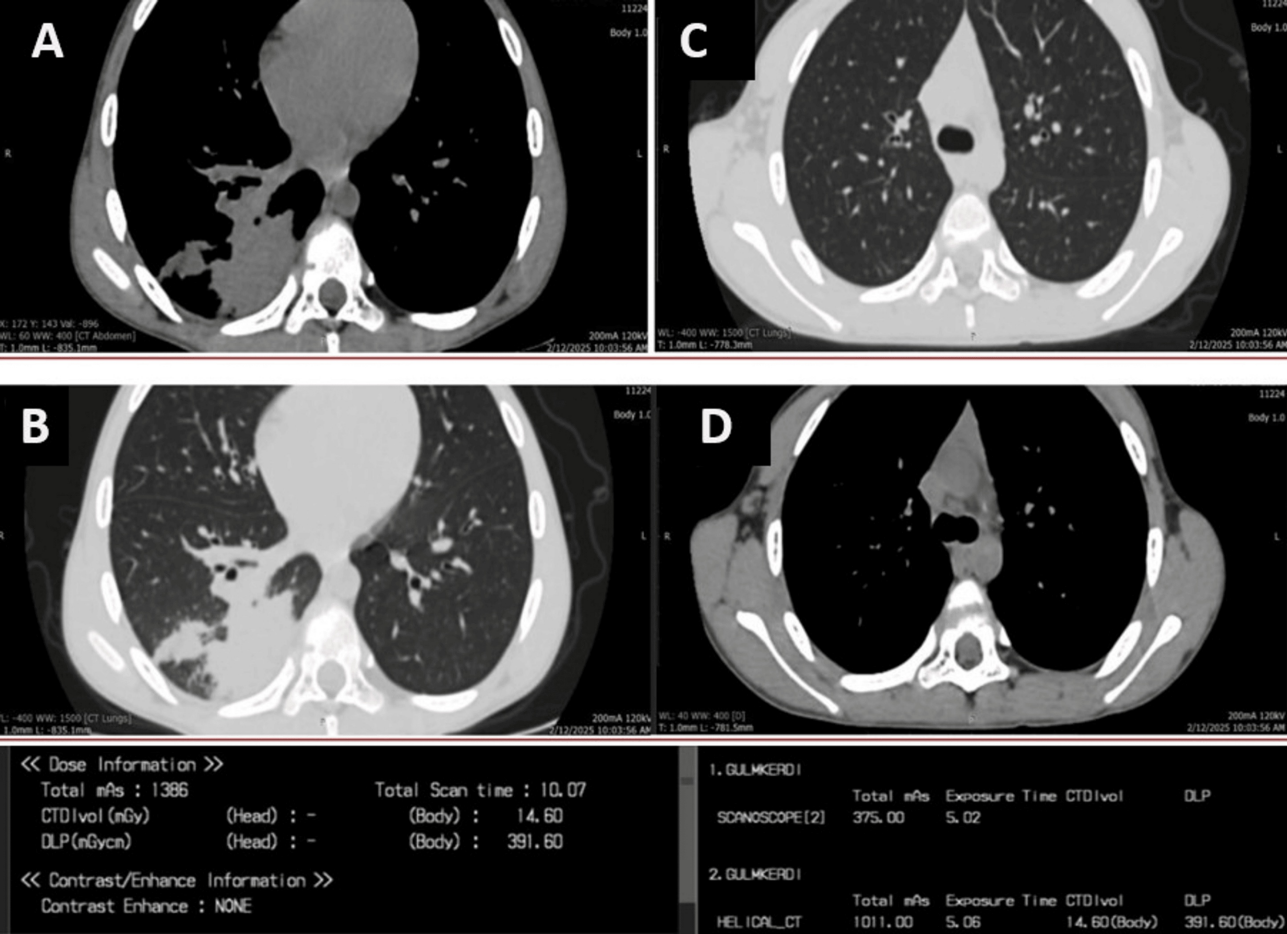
Pediatric chest CT scan showing clinical pathology. (A) Axial soft tissue window and (B) lung tissue window. Consolidative and infiltrative changes are visualized in the right lung despite lower dose parameters. (C) Axial lung window and (D) axial soft tissue window, no pathological changes.
